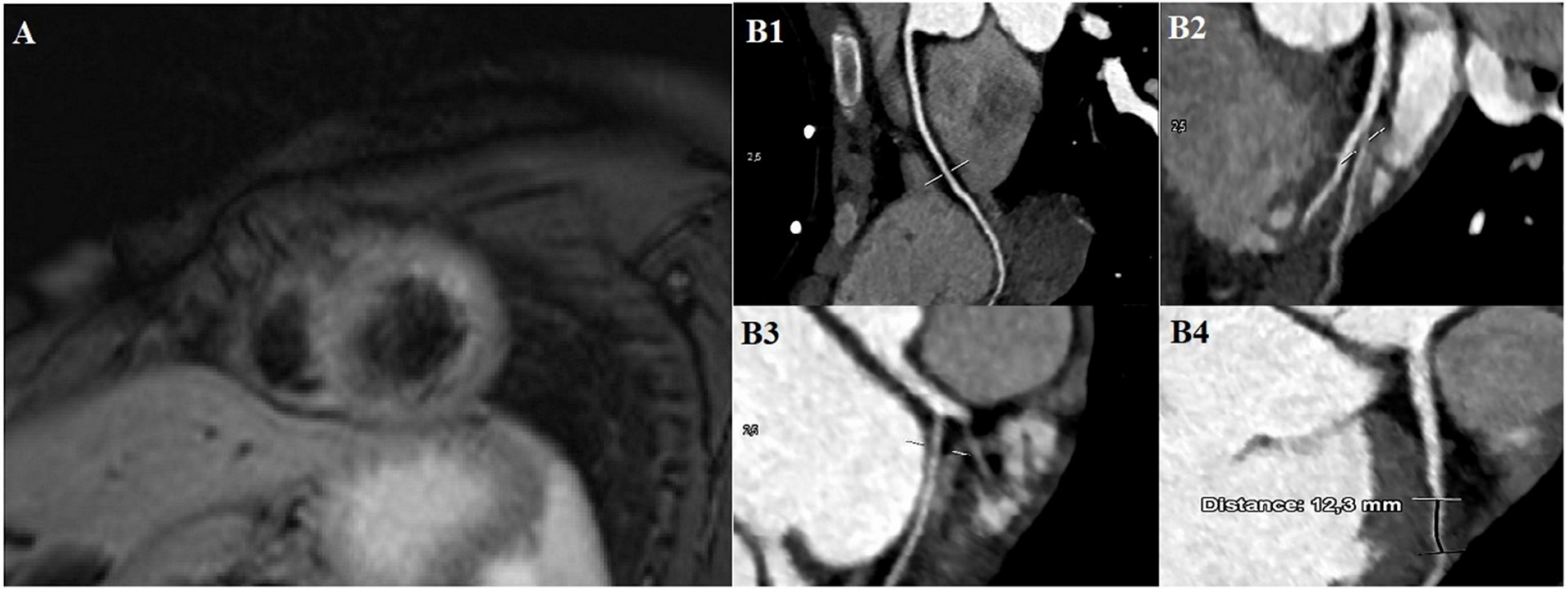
(A) Cardiac contrast-enhancement magnetic resonance imaging demonstrates an increase of T2 signal on anterior left ventricular wall. (B1–B4) Coronary computed tomography reveals a right dominant coronary artery (B1), left common trunk coronary artery (B2), left circumflex coronary artery (B3) and left anterior descending (LAD) coronary artery (B4) without any dissection, lumen obstruction or anatomical variants. A tract of LAD coronary artery runs through the width of the myocardial wall for 12.3 mm and with a parietal thickness of 1.4 mm (B4).
